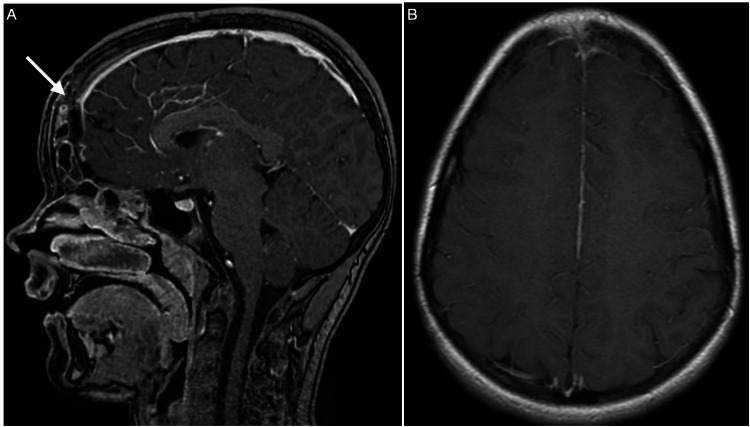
Postoperative MRI findings after Pott’s puffy tumor treatment. Six-month MRI showing the epidural collection had completely drained, and the patency of the superior sagittal sinus remained. Small bone continuity is observed (arrow) (A), and frontal convexity is regular in shape (B).
